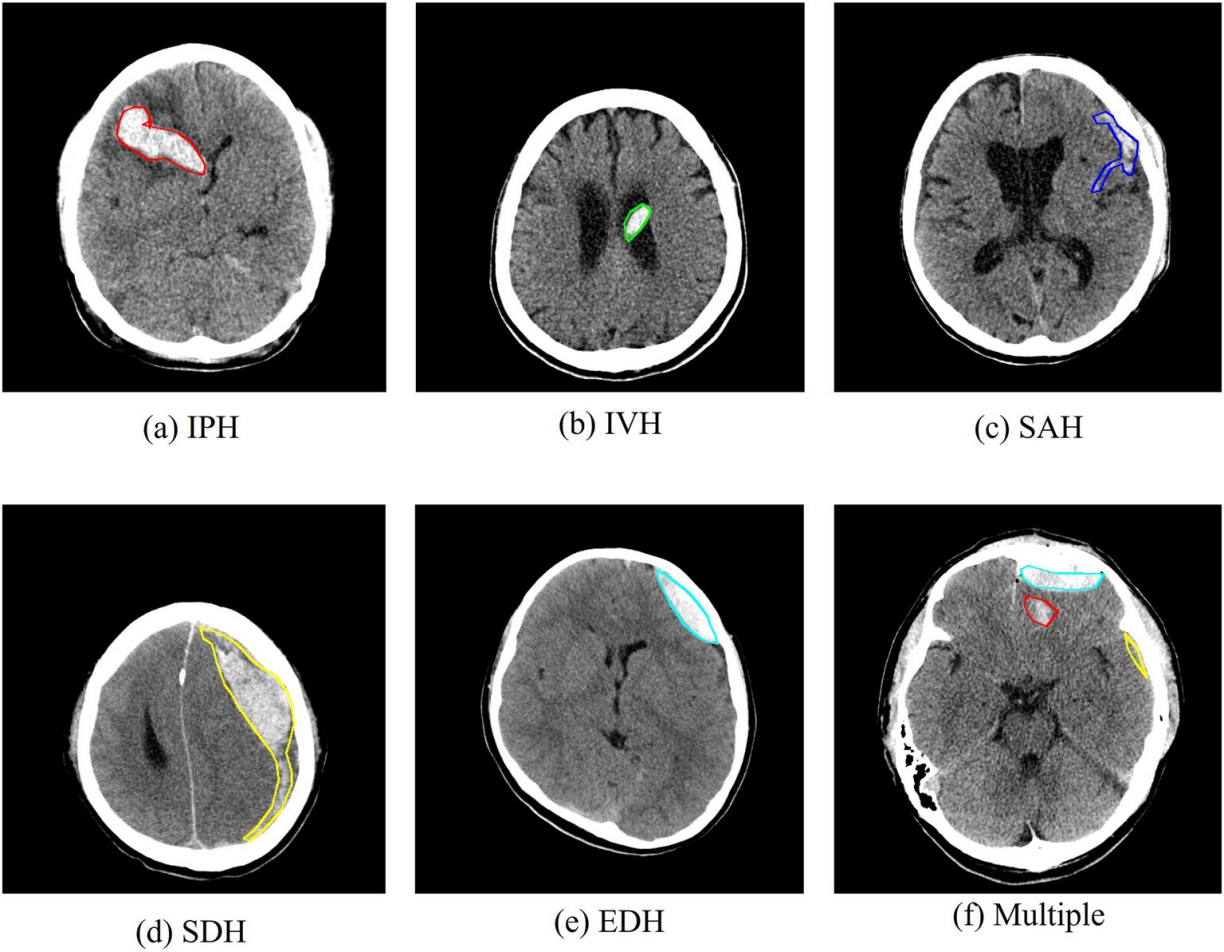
Data examples; (a) example of intraparenchymal hemorrhage (IPH); (b) example of intraventricular hemorrhage (IVH); (c) example of subarachnoid hemorrhage (SAH); (d) example of subdural hemorrhage (SDH); (e) example of epidural hemorrhage (EDH); (f) CT images with one or more cerebral hemorrhagic lesions (the image include IPH, SDH, and EDH).
